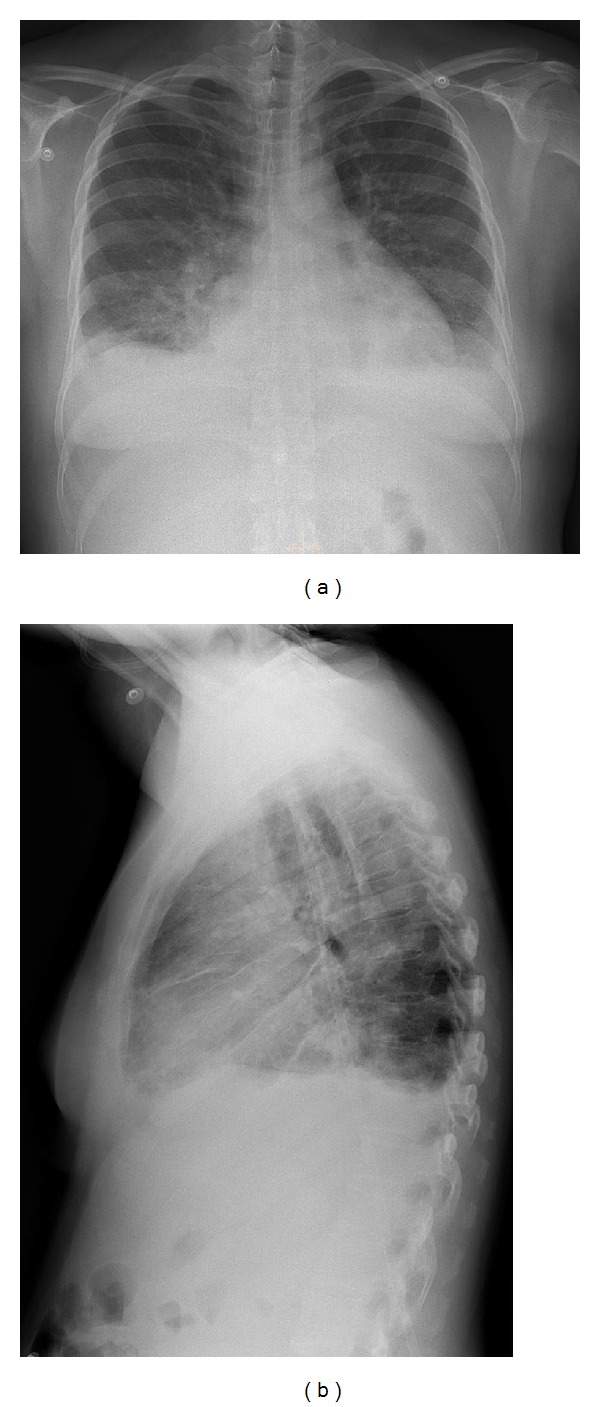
A 42-year-old female with hypertrophic cardiomyopathy and acute pulmonary edema. Findings: chest radiographs (PA (a) and lateral (b)) demonstrate small bilateral pleural effusions, cardiomegaly, and perihilar airspace opacities in keeping with pulmonary edema.
